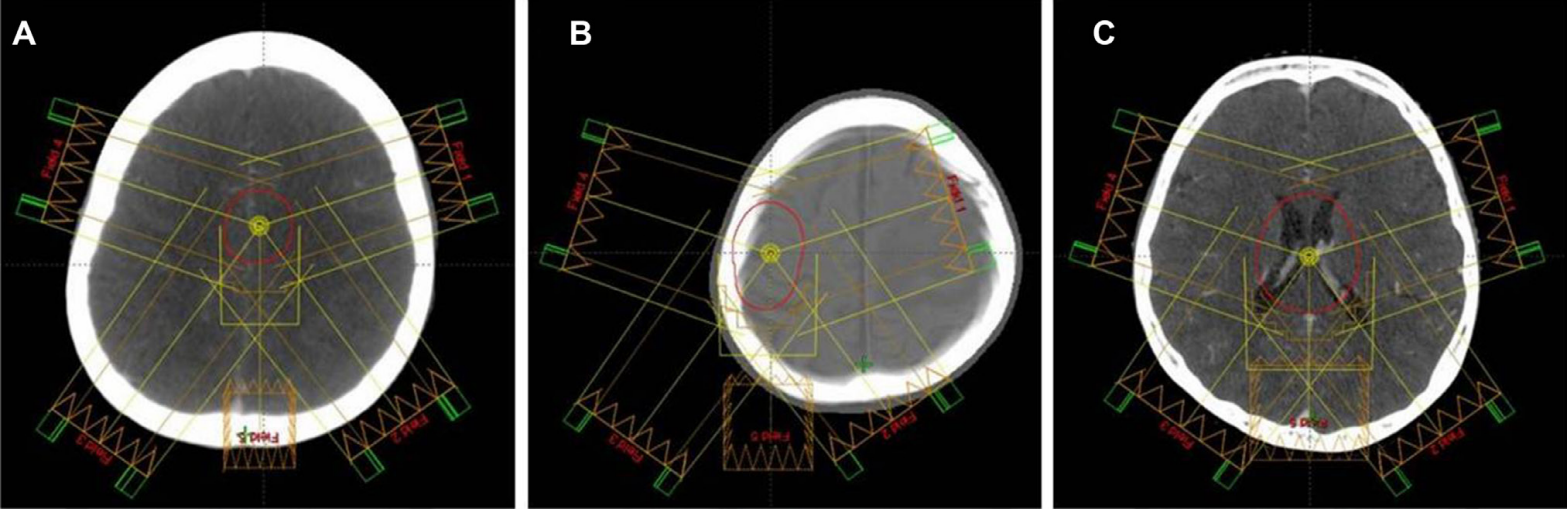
Typical target volumes delineated on pseudo-CT scans: (A) PTV_ord_, (B) PTV_skull_, and (C) PTV_vent_. In each figure the field alignment in each treatment plan is shown.
